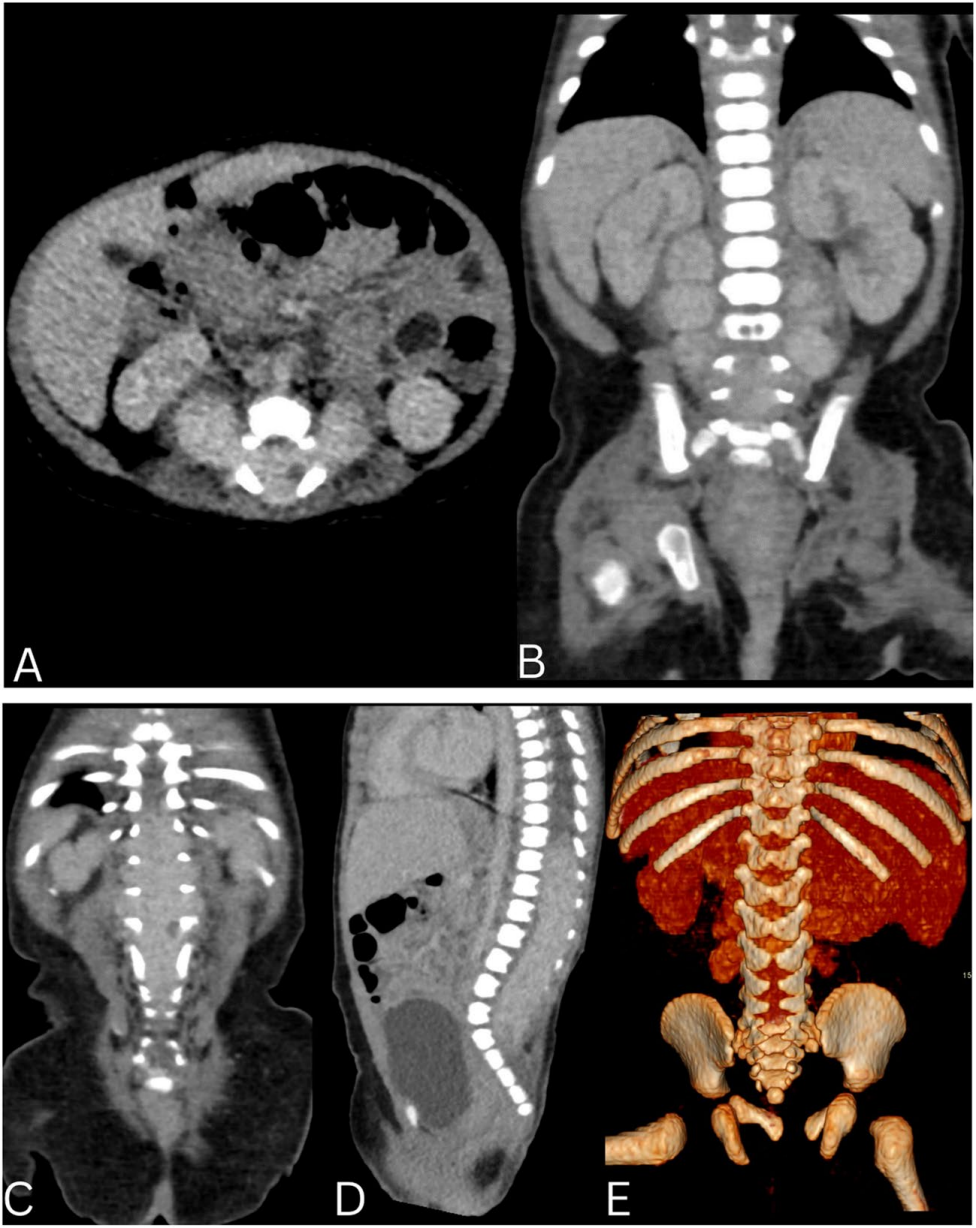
Axial (image A), Coronal (image C and D) contrast enhanced CT scan of the spine with 3D reconstruction (image E) showing a high attenuation mass occupying the lower thoracic and lumber spinal canal, extending through multiple neural foramina forming a Dumble shaped appearance (image A). 3D reconstruction images show multilevel posterior elements failing to fuse in keeping with Spina bifida (image E)
